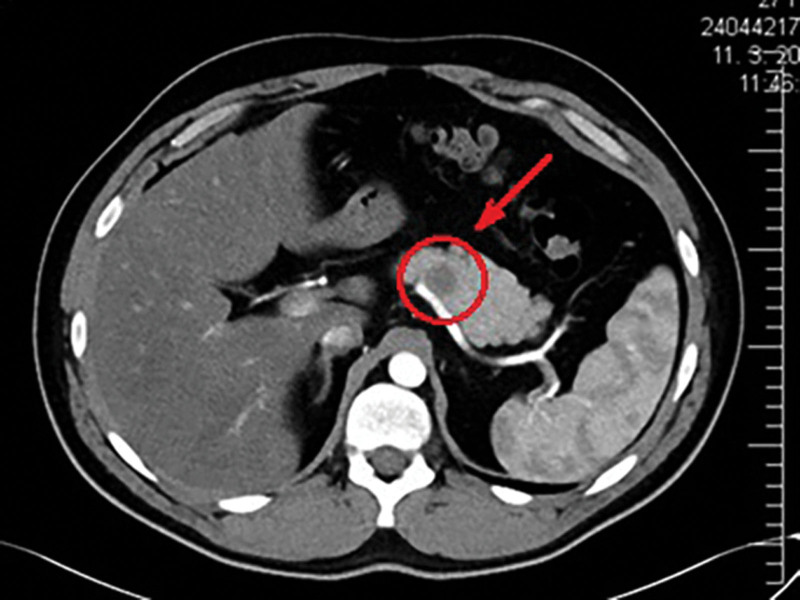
Computed tomography in the axial plane. The red arrow indicates the pancreatic lesion. Source: Authors’ archive, Department of Surgery, Faculty Hospital Nitra, Nitra, Slovakia.
